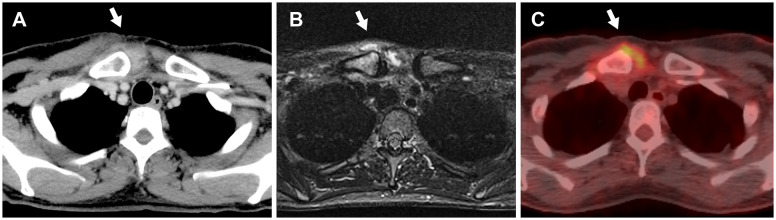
The imaging findings of the right sternoclavicular joint without evidence of bone fractures, abscesses, osteonecrosis, or metastasis (white arrows). (A) Computed tomography image shows increased fatty tissue concentration. (B) Magnetic resonance imaging with short tau inversion recovery reveals hyperintensities. (C)
